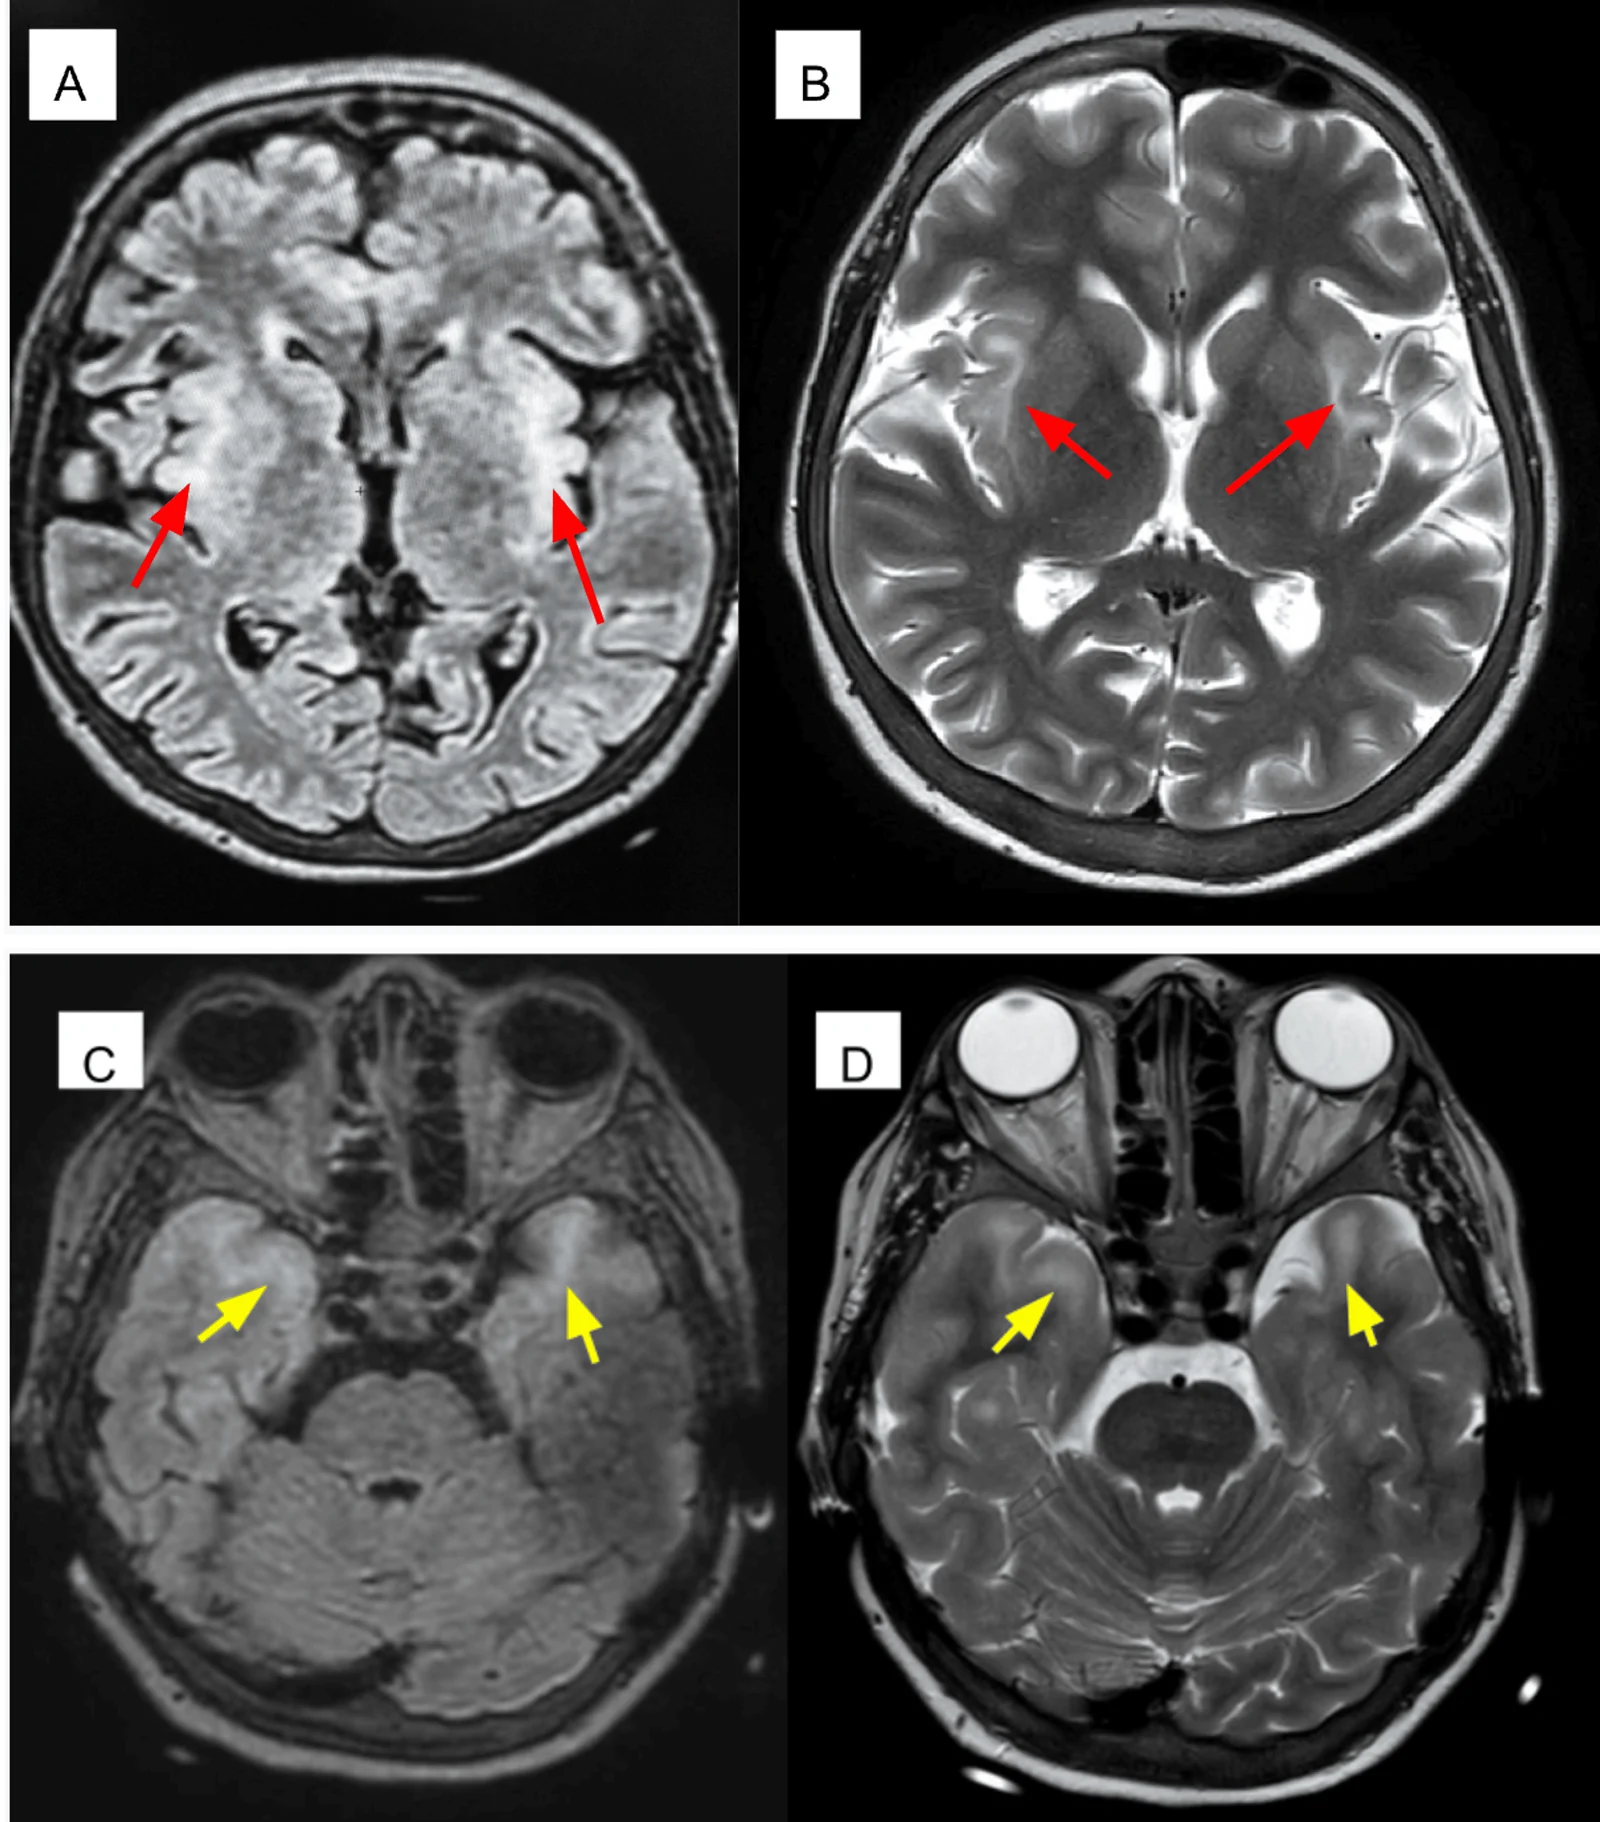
Initial brain magnetic resonance imaging (MRI) of a 23-year-old female patient Axial fluid-attenuated inversion recovery (FLAIR) (A, C) and T2-weighted (B, D) images of the hemisphere. There were hyperintense lesions in the insular and temporal areas consistent with vasogenic edema (red arrows). There were also hyperintensities in both amygdalae (yellow arrows).
